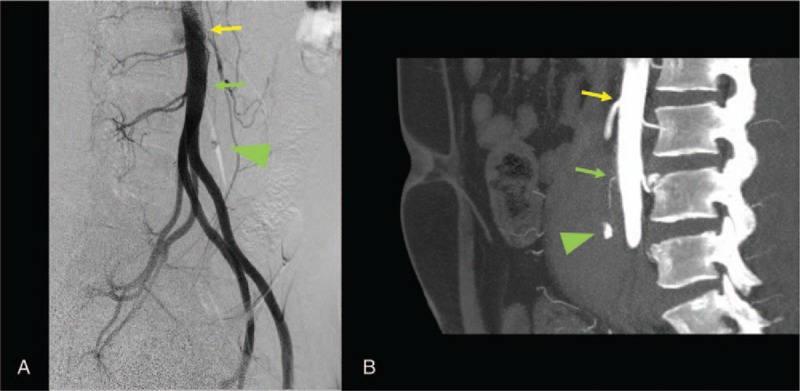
Right oblique image of aortography (A) and left lateral image of CT aortography (B) 6 hours after injury showing contrast extravasation (triangle arrow). The affected vessel arose from the anterior wall of the abdominal aorta (yellow arrow) 41 mm below the origin of the inferior mesenteric artery (green arrow). CT = computed tomography.
